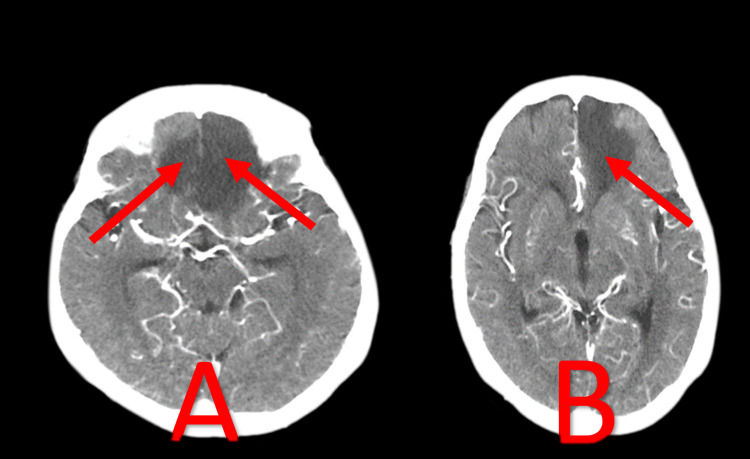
CT brain on the 2nd day post admission Bilateral frontal ischemia. Image A showed diffuse hypo-dense lesions involving the parasagittal part of the right and left frontal lobes (red arrows). Image B showed hypo-dense lesions involving the parasagittal part of the left frontal lobe
